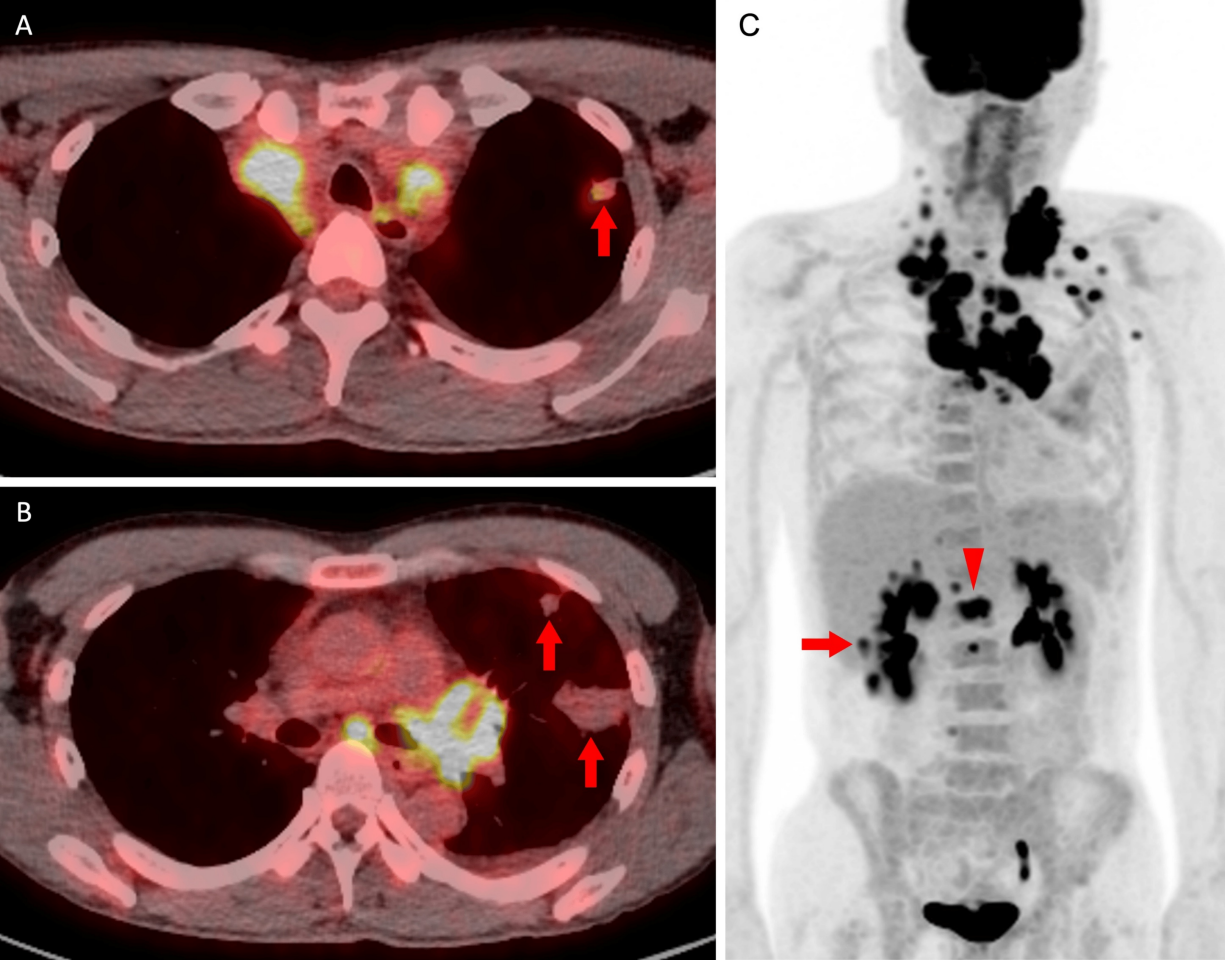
18F-FDG PET/CT findings 18F-FDG PET/CT shows intense FDG uptake in the enlarged lymph nodes (A-C) and moderate uptake in the pulmonary nodule (A, arrow), right kidney (C, arrow), and lumbar vertebrae (C, arrowhead), while no abnormal FDG accumulation is observed in the wedge-shaped opacities in the left lung (B, arrows). FDG: fluorodeoxyglucose
